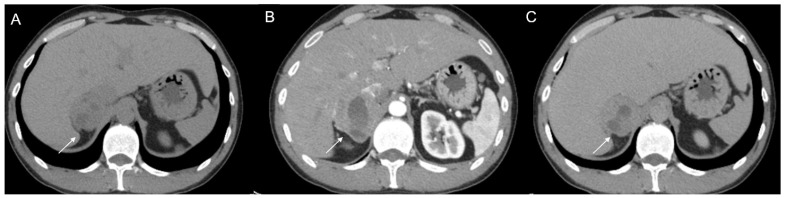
36-year-old man with history of night sweats, hypertension, and tachycardia due to pheochromocytoma (arrow). Non-contrast CT shows an inhomogeneous right adrenal mass > 20 HU (A). In the arterial phase (B), the mass shows intense enhancement of the solid components with persistent enhancement. In the late phase (C), absolute contrast medium washout is <50%.
